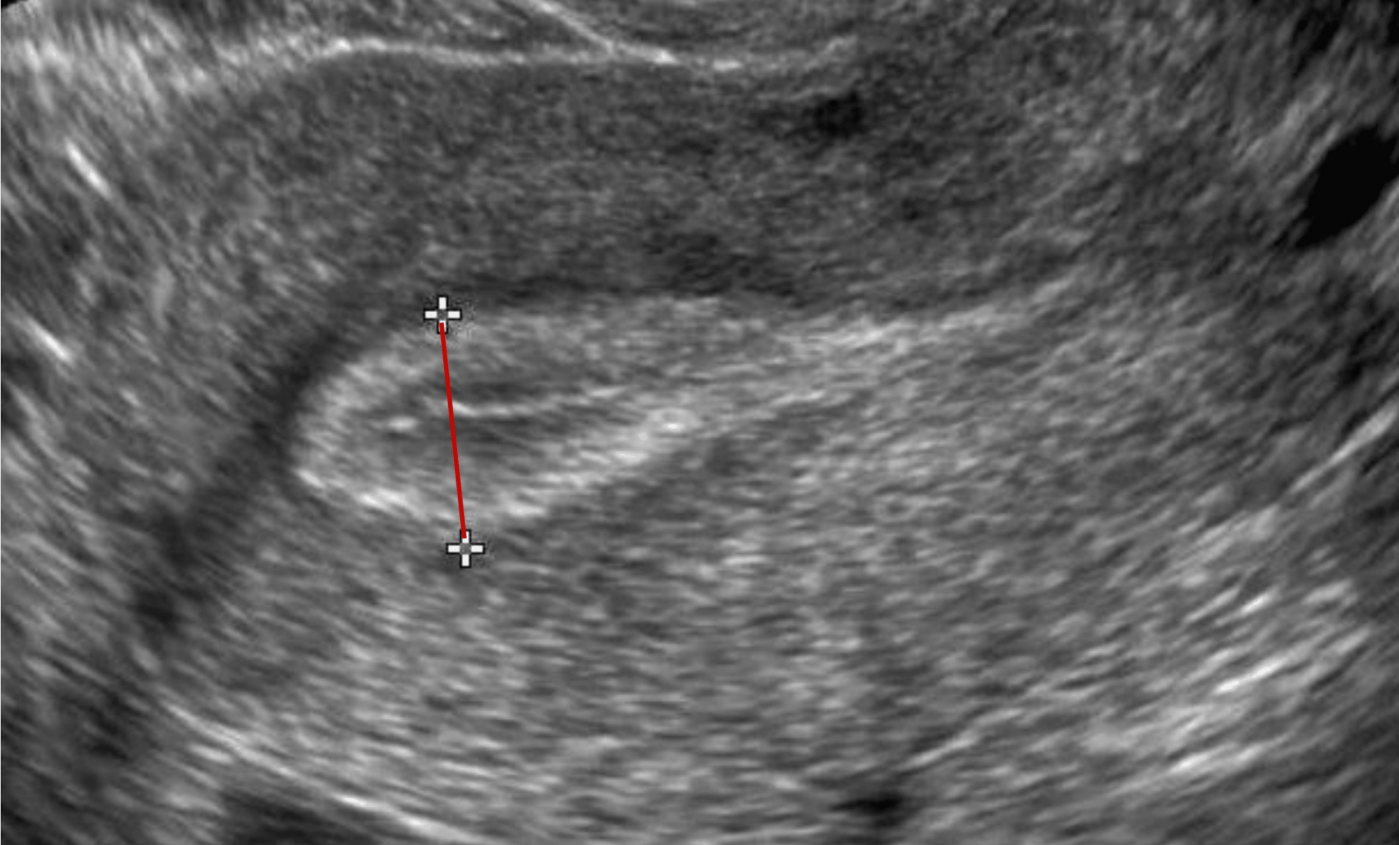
Ultrasound showing the measurement technique for EMT. EMT: endomterial thickness.
